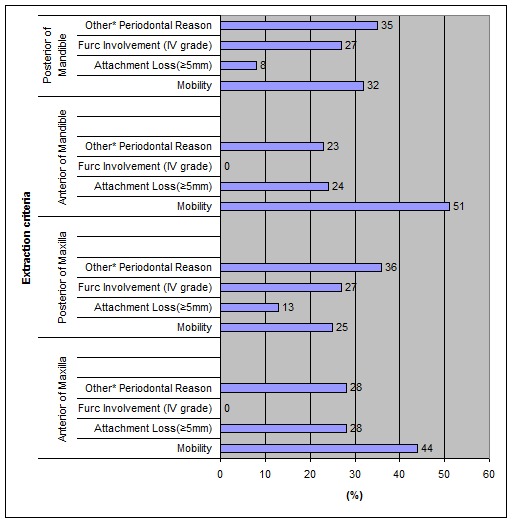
Distribution (%) of teeth extracted due to periodontal reasons solely (extraction criteria by Moreira et al.) *Other periodontal reasons include: radiographic bone loss (RBL) greater than 50% prosthetic planning, socio-economic and cultural aspects (Moreira et al.)
